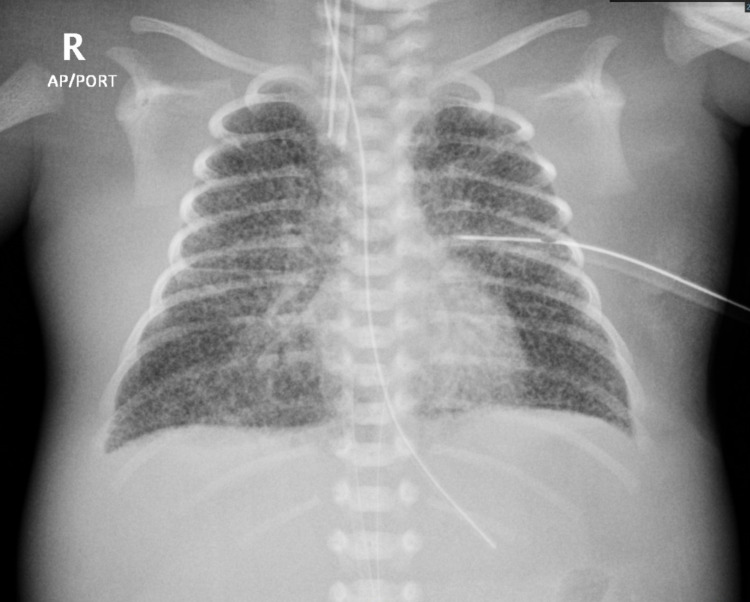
Chest radiography showed resolving pneumothorax after the insertion of left chest tube with re-expansion of the partially collapsed left lung.
